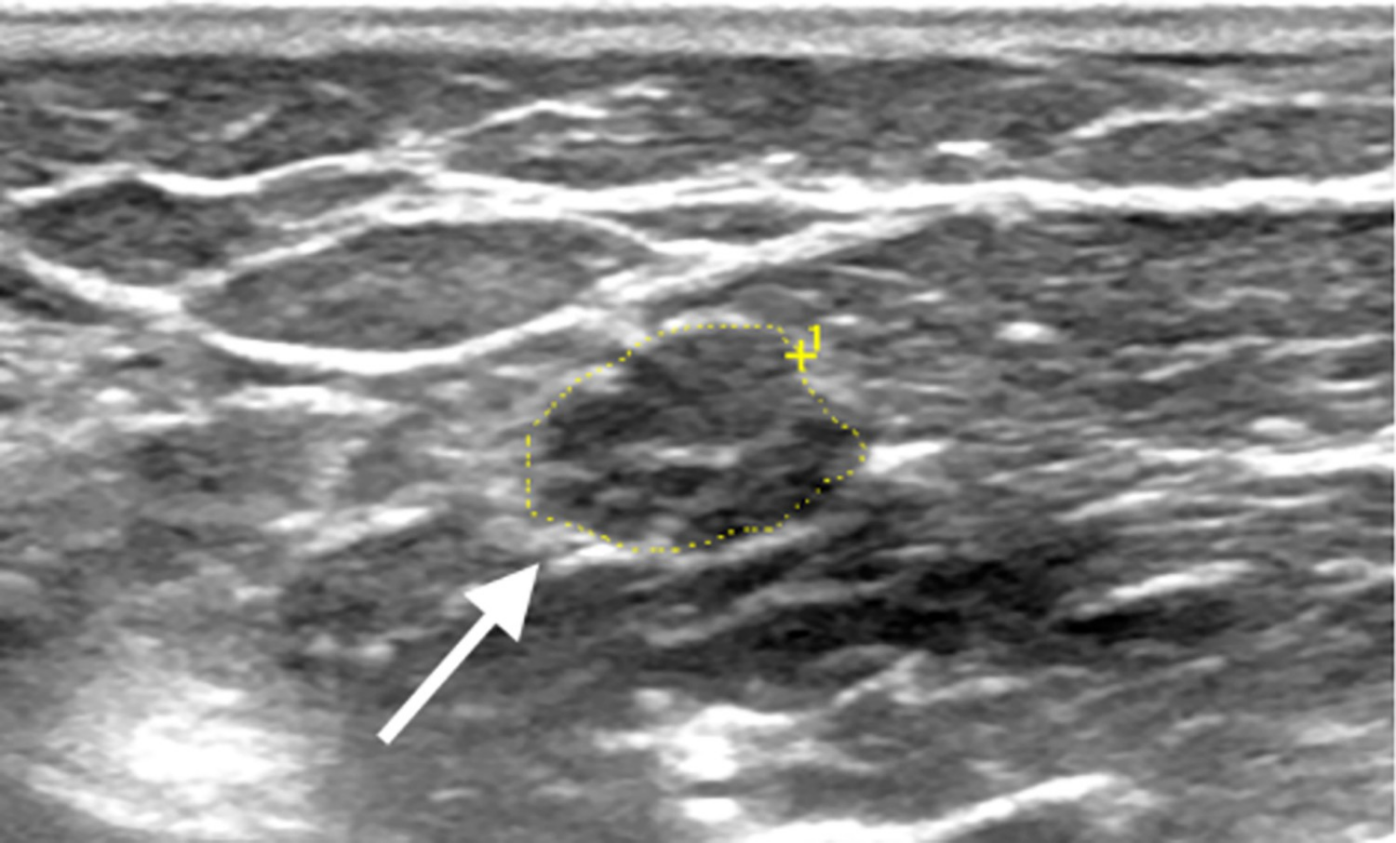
Ultrasound of the ulnar nerve (transverse view) proximal to the cubital tunnel in a leprosy patient, showing neural thickening and enlarged fascicles (white arrow).
